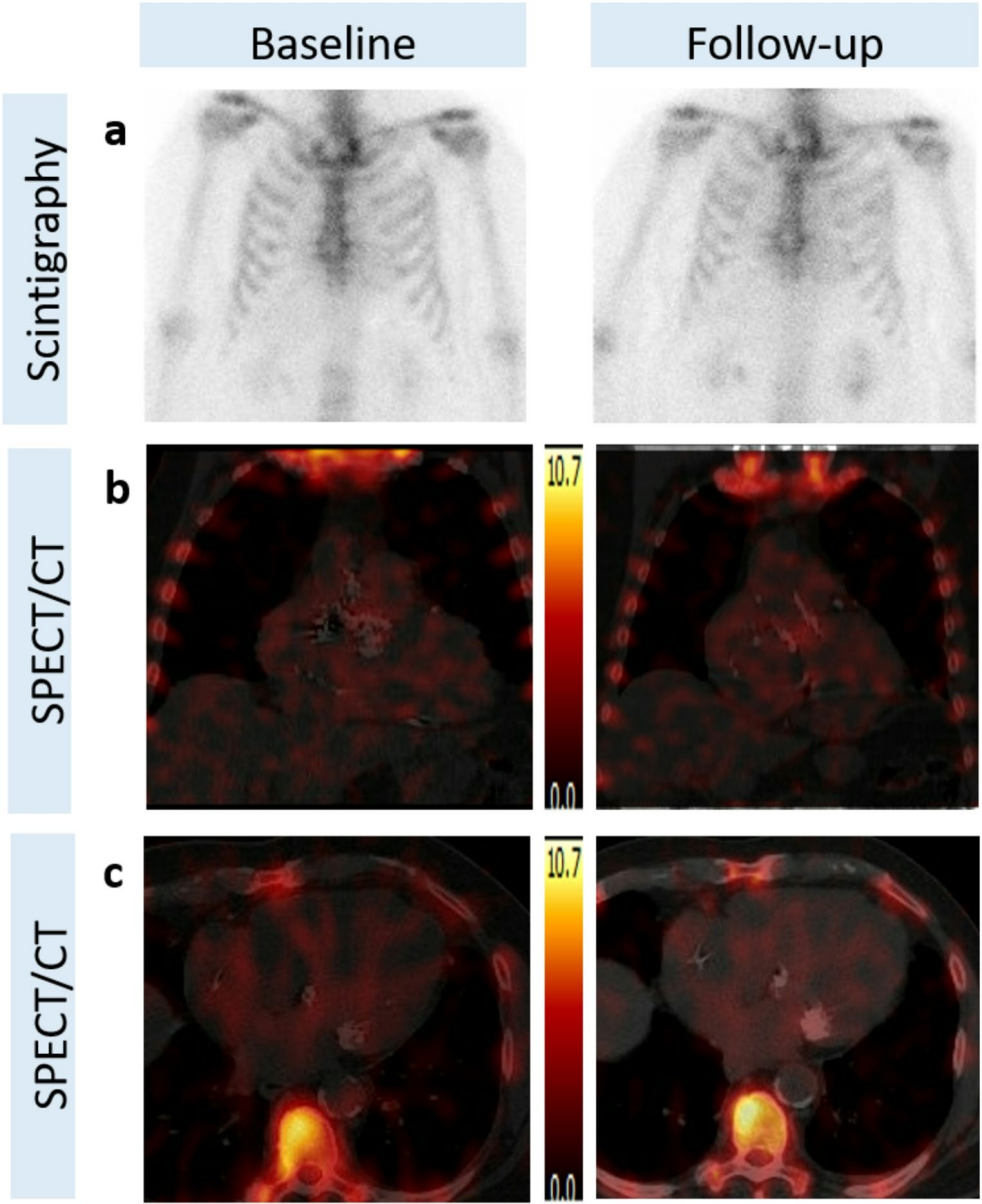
Representative scintigraphic images (a) of a patient with Perugini grade 1 at baseline (left) and at follow-up (right). SPECT/CT depicts the absence of LV uptake on the coronal (b) as well as on the transaxial (c) images in both examinations, baseline (left) and at follow-up (right), respectively
